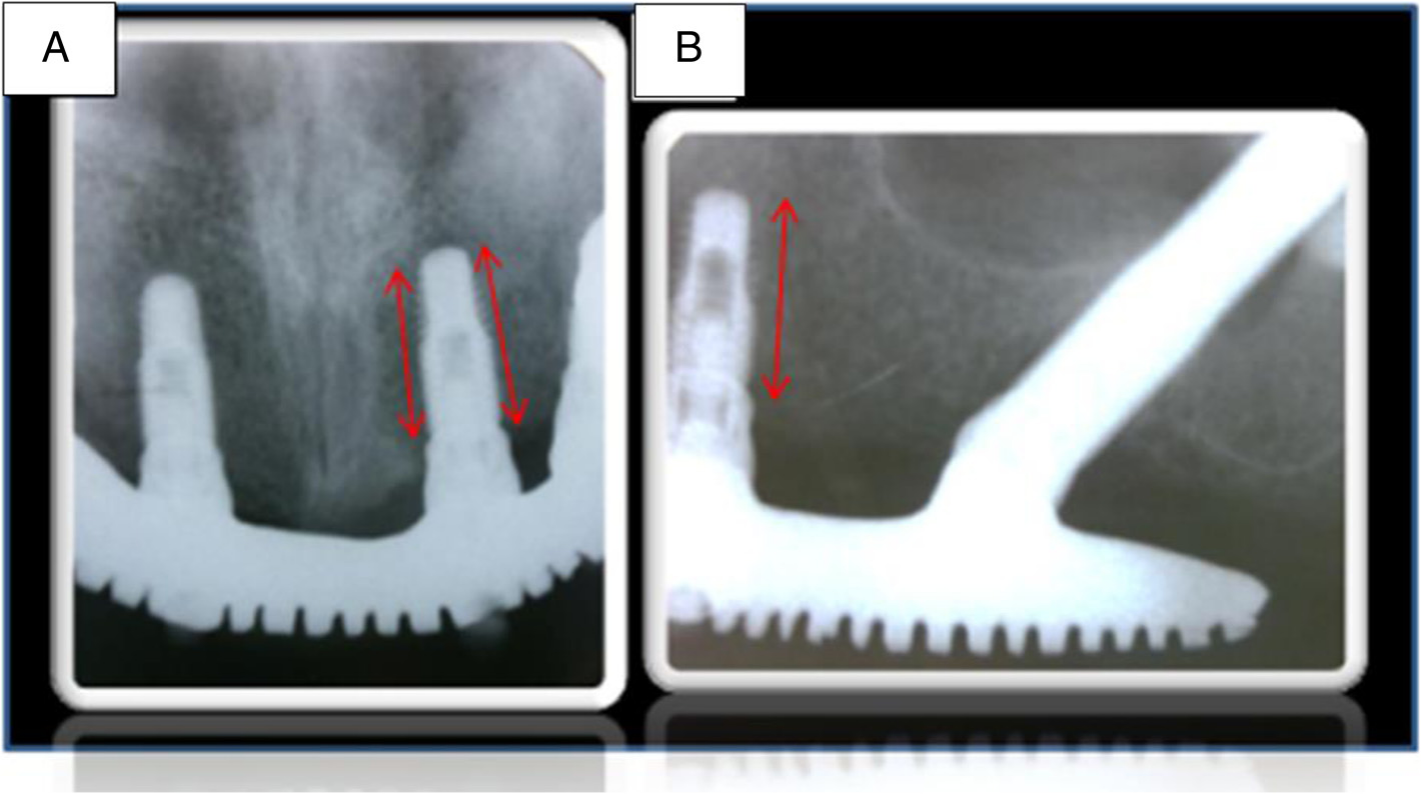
Periapical radiographs using the parallelism technique. a Conventional implants—anterior. b Conventional implants—posterior
